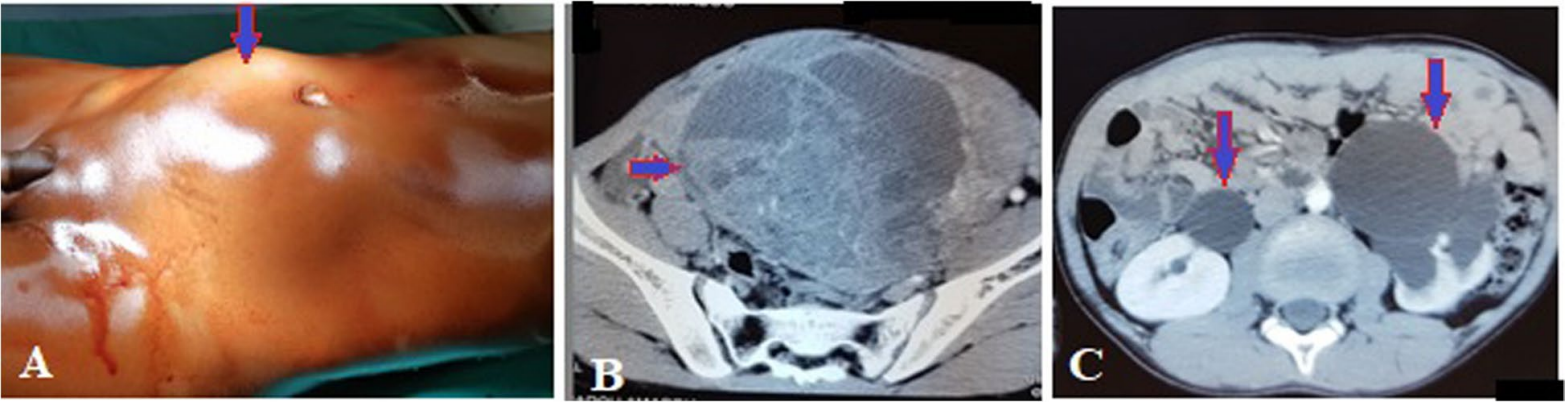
Pelvic mass in a 12-year-old child (A): represented by the axial slice CT image (B) of an irregular pelvic mass with tissue and fluid content, resulting in a bilateral compression hydronephrosis uretero (C blue fleches)
